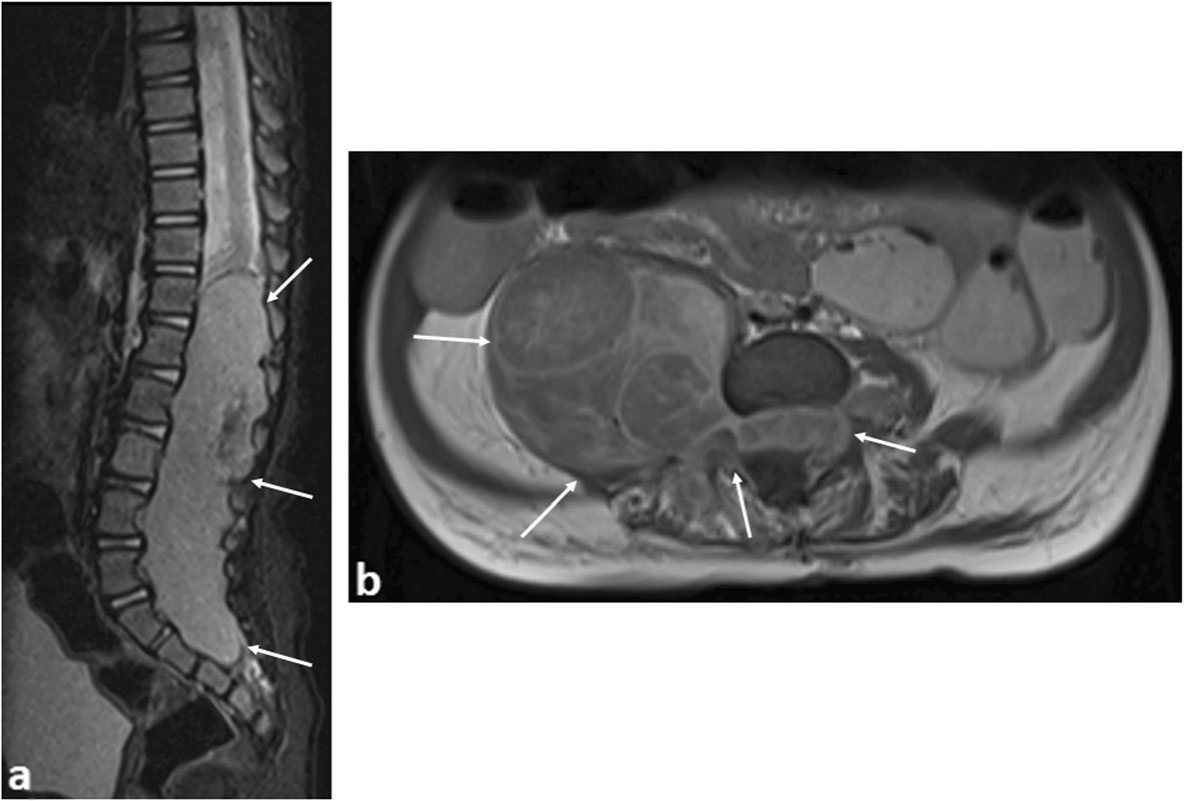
A 9-month-old boy with paravertebral dumbbell neuroblastoma. a Sagittal T2-weighted fat-saturated MR image shows a large soft tissue mass occupying and expanding the spinal canal from T12 to S3 levels (arrows), also causing compression of the spinal cord. b Axial T1 post-contrast imaging reveals a large right paravertebral mass (arrows) with intraspinal extension, occupying the entire right-sided neural foramen. Urgent spinal laminectomy and decompression was subsequently performed
